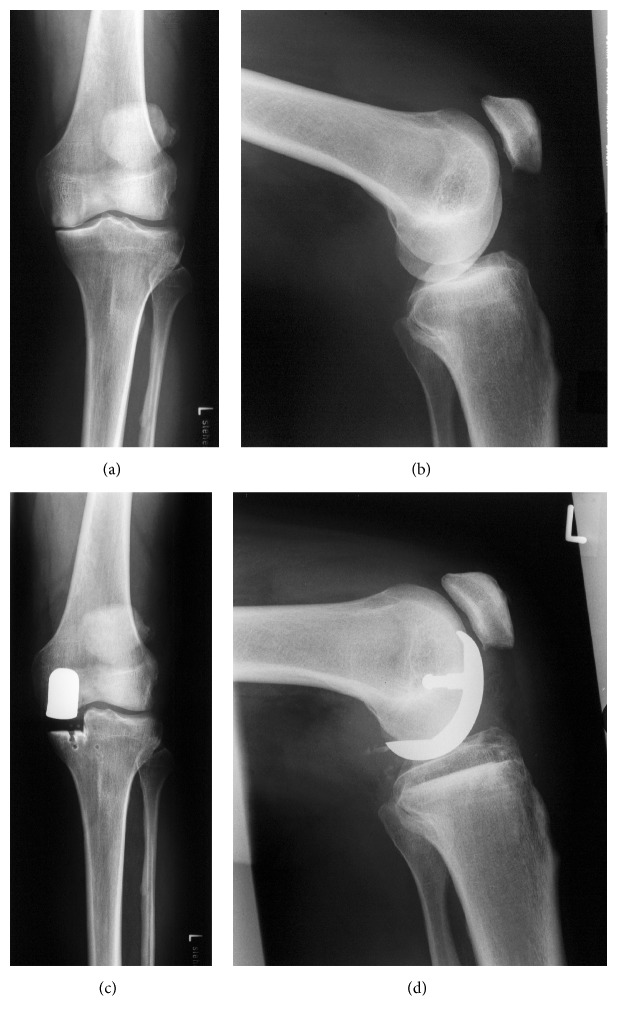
Unicompartmental knee (Preservation, DePuy, Warsaw, IN, USA), pre- and postoperative AP and lateral radiographs.
